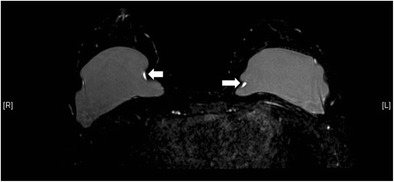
Periprosthetic fluid. T2-weighted fat-saturated axial MR image shows trace amount of periprosthetic fluid bilaterally (arrows), which is a common normal finding
